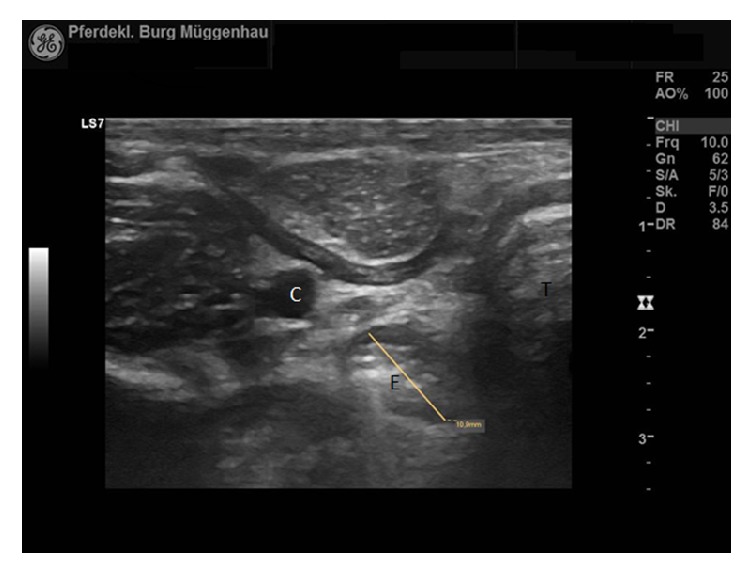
Ultrasound image of the stricture site at final follow-up examination. Diameter was measured at 10,9 mm. Left in the image is dorsal. C: carotid artery. E: esophagus. T: trachea (probe frequency 10 MHz, depth 3,5 cm).
